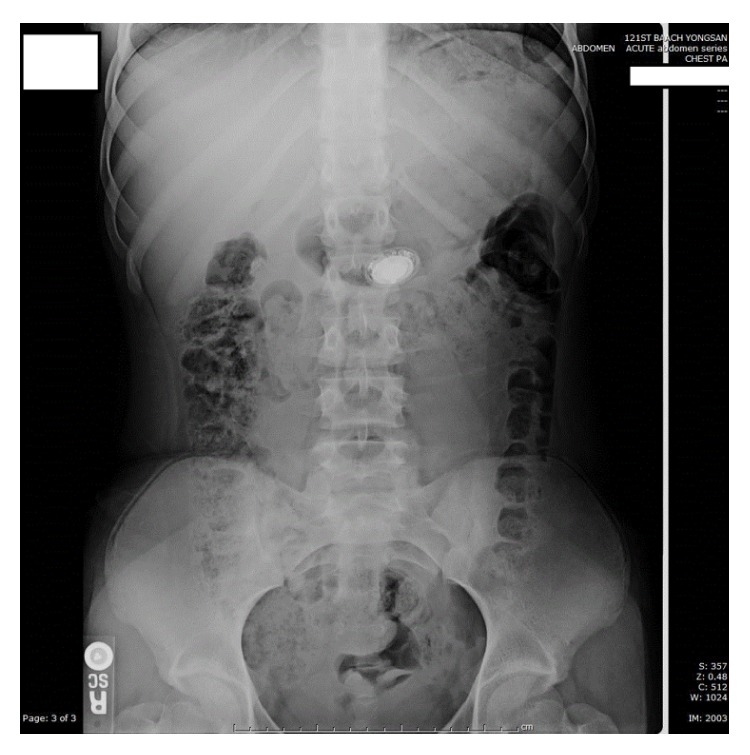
Supine abdominal X-ray showing watch in the stomach.
